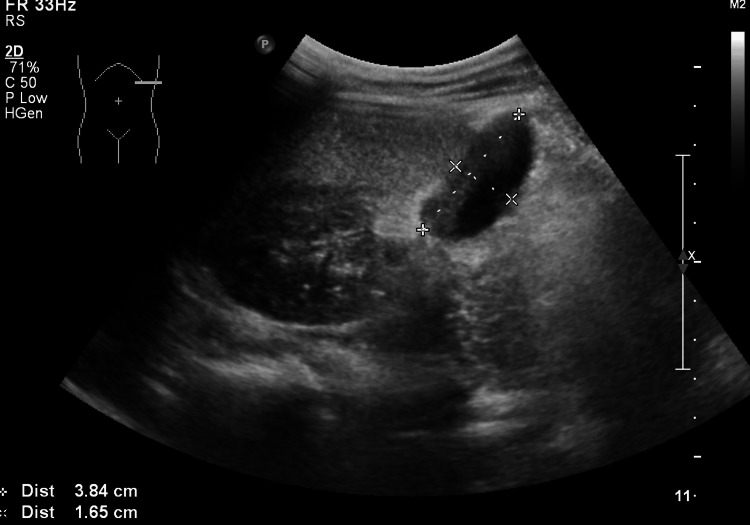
Abdominal ultrasound demonstrating a cyst located in the pancreatic tail.
